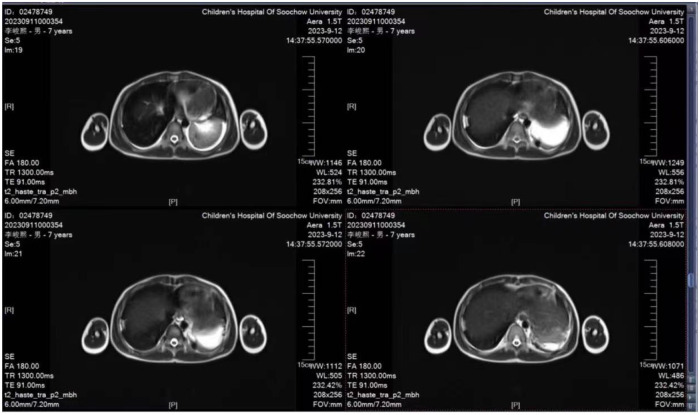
Chest MRI (2023-09-12) showed large pressure pressure hyperintensity in the left lower lung area and flocculate pressure hyperintensity in the right lobe of the lower lung.
